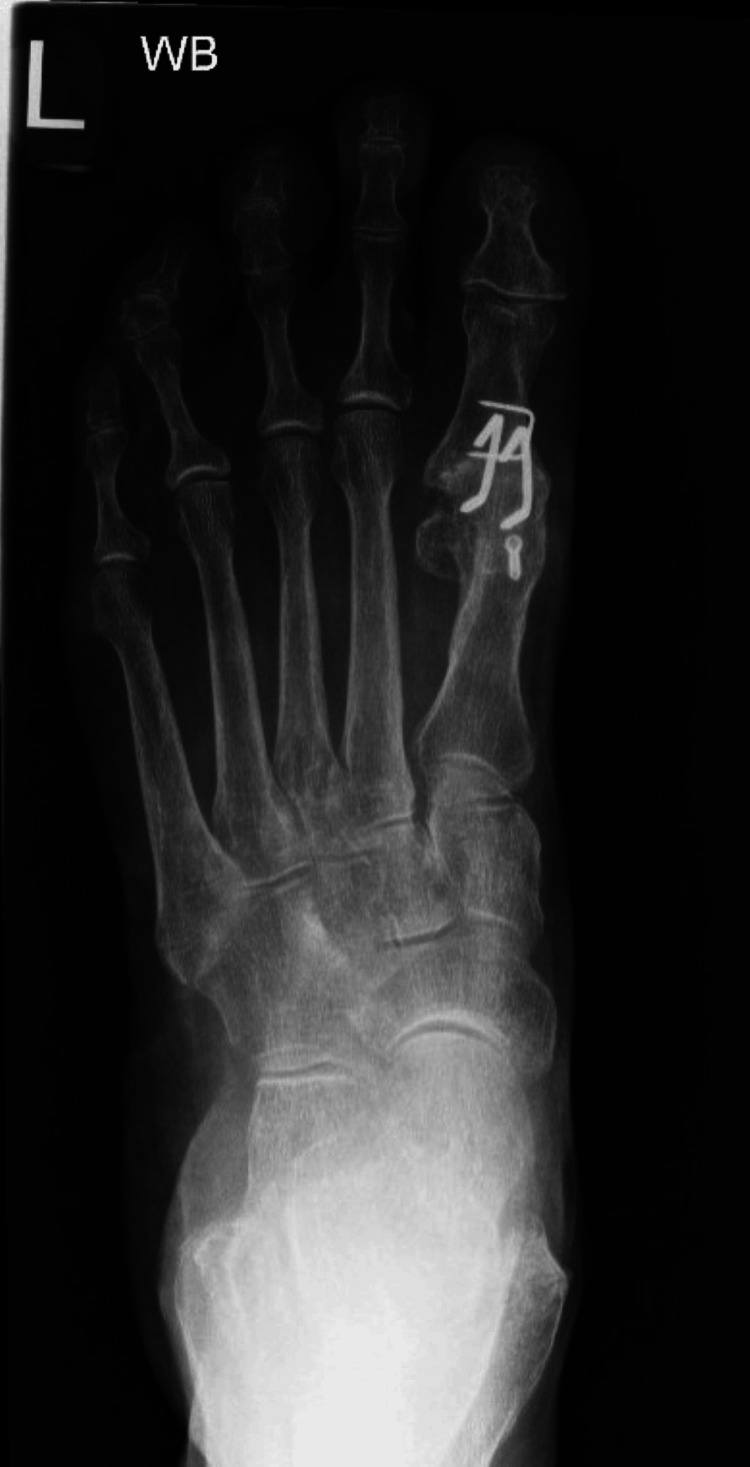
AP X-ray of left foot taken at six weeks showing signs of healing WB: Weight-bearing; AP: Anteroposterior
